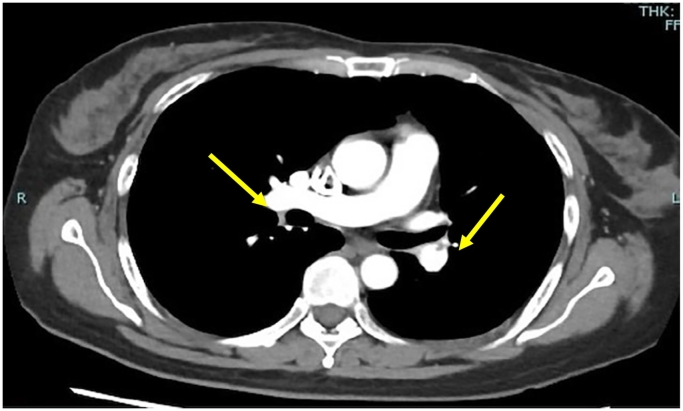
Computed tomography on the 8th day of hospitalization. The thrombi have disappeared.
